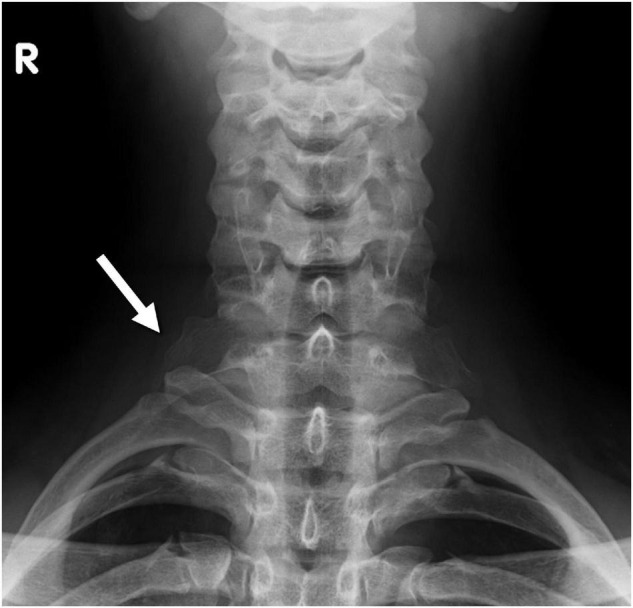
Radiograph of the cervical spine shows a stump rib on both sides (white arrow).
